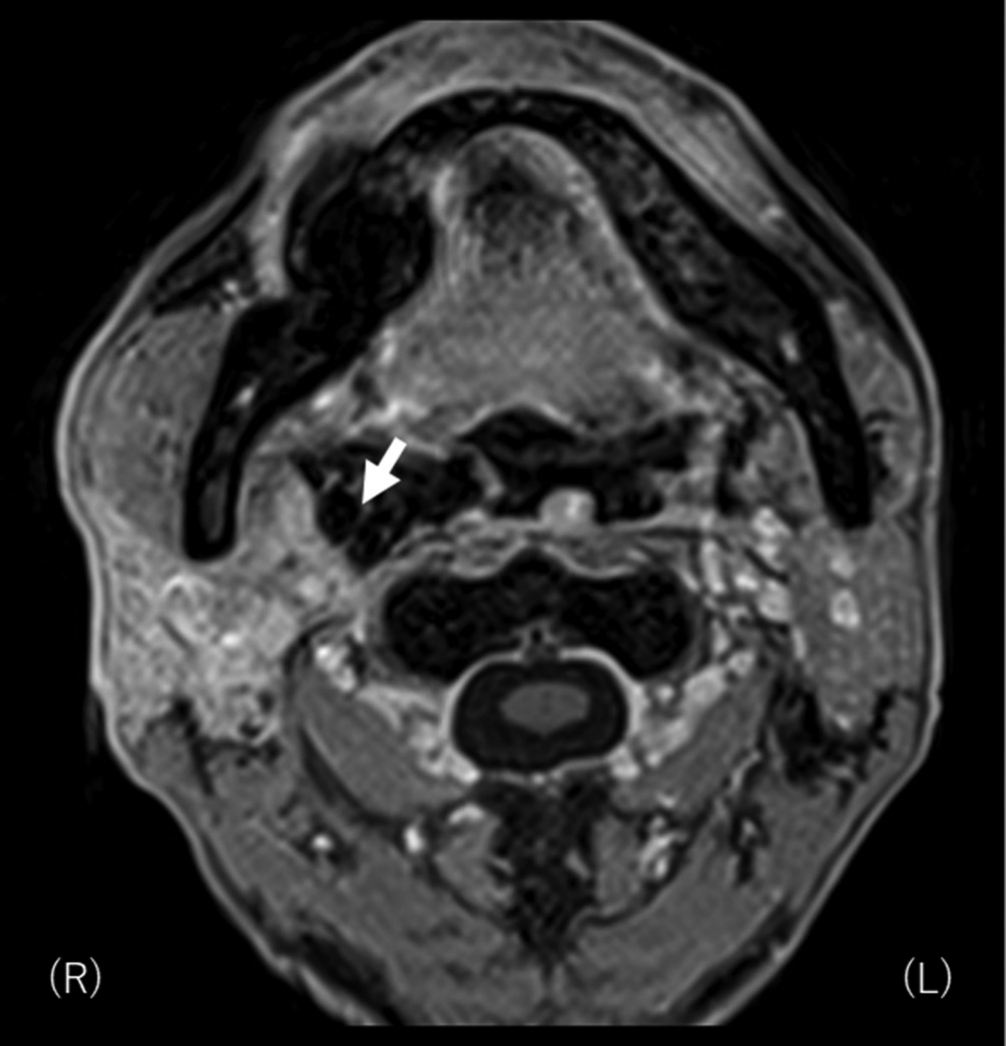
MR image was taken 2 months after interstitial brachytherapy. No evident residual tumor was found beneath the ALT flap. ALT, anterolateral thigh.
